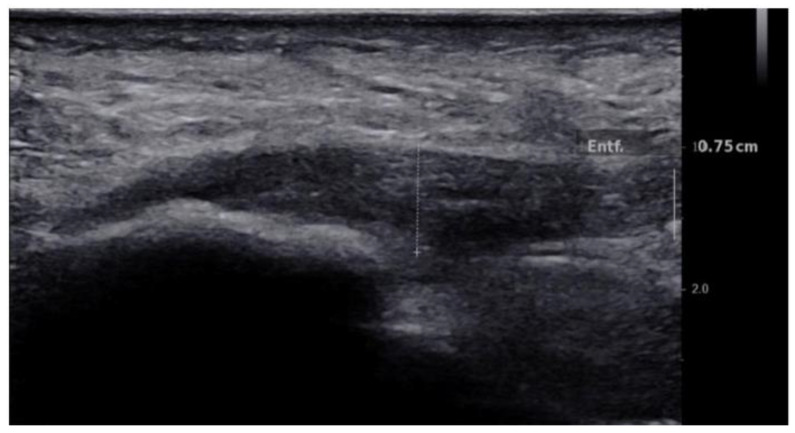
Longitudinal B-mode ultrasound image of a 50-year-old patient with plantar fascia shows hypoechogenicity and thickening of the plantar fascia of 7 mm.
